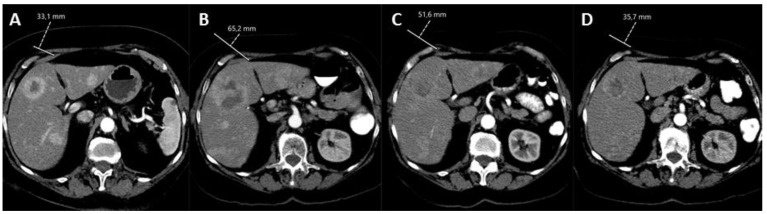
Patient with a grade 1 pNET with liver metastases, who participated in the HEPAR PLuS trial. (A) Baseline CT prior to PRRT with 4 cycles of 7.4 GBq ^177^Lu-DOTATATE. (B) CT 3 months after PRRT depicting evident progressive intrahepatic disease. (C) CT 3 months after additional ^166^Ho-radioembolization showing increased necrosis, size reduction and reduced enhancement of neuroendocrine liver metastases, and stable disease according to RECIST 1.1 (−22%). (D) CT 12 months after additional ^166^Ho-radioembolization showing advancing partial response according to RECIST 1.1 (−44%) and tumor reduction.
